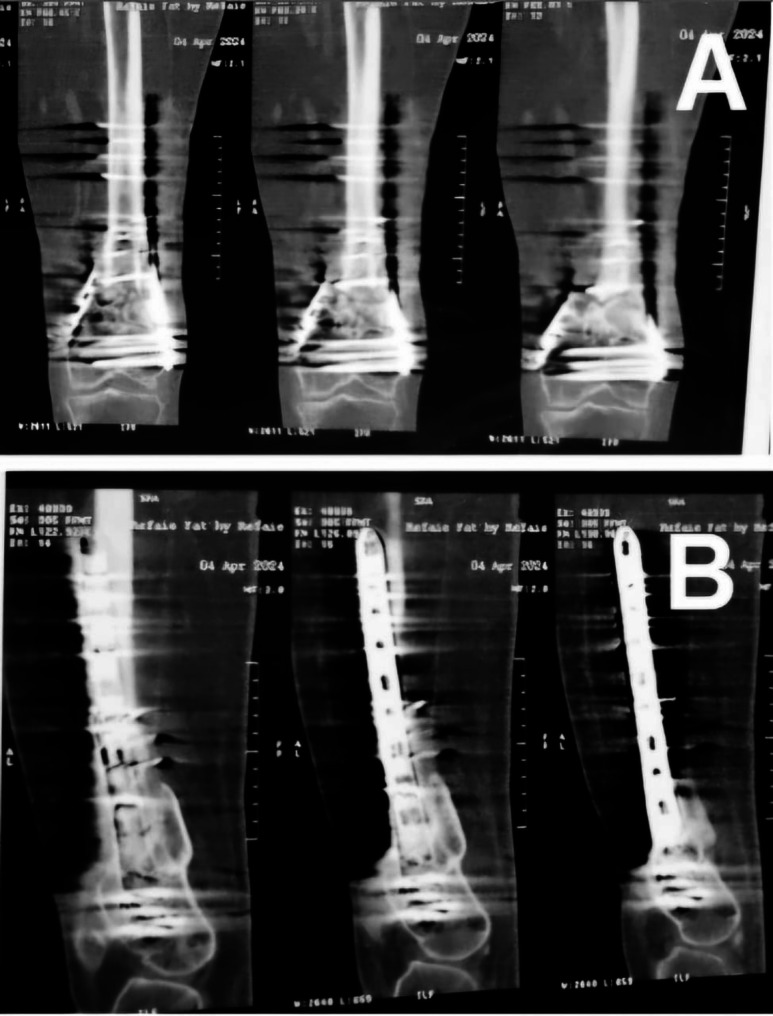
CT scan of the distal femur showing fracture union in both the coronal (A) and sagittal (B) views
